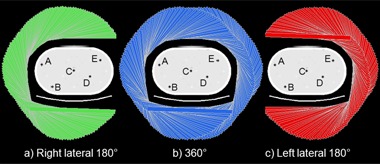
Three detector trajectories were each used to acquire 10 independent, 4.5 min SPECT scans.
